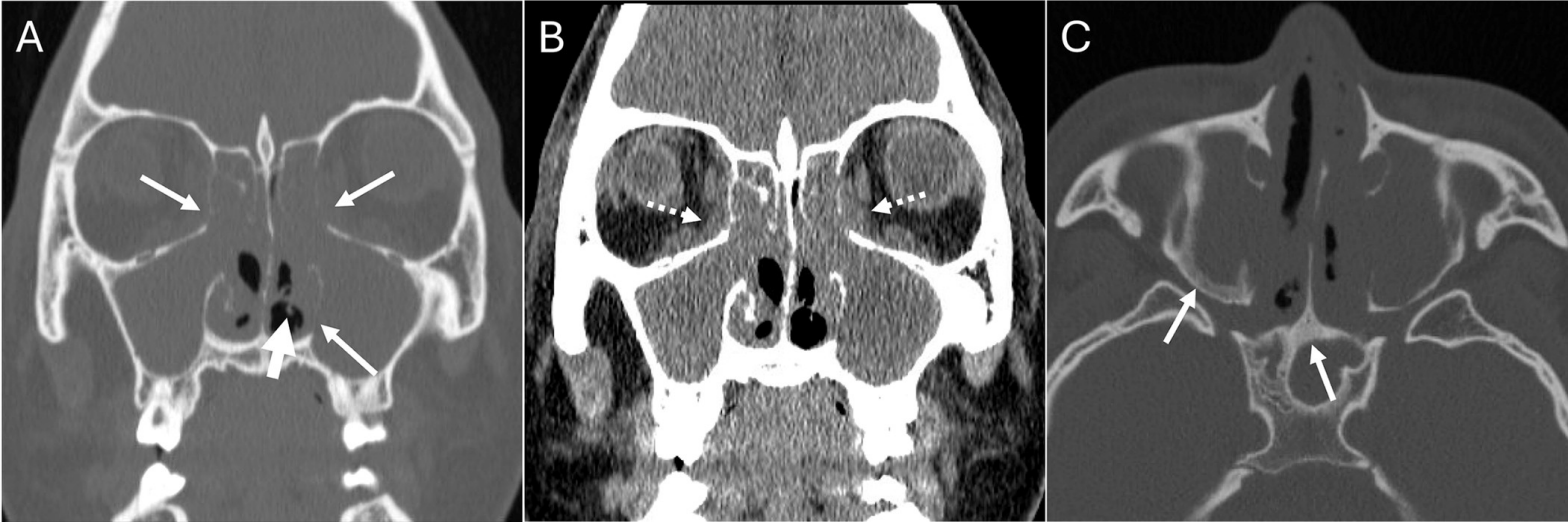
Granulomatosis with polyangiitis (GPA). Coronal bone (A) and soft tissue (B) window non-contrast CT of the sinuses in a 22 year-old male shows typical pan-sinus soft tissue opacification and nasal strands (thick arrow, A), foci of osseous erosion (thin arrows, A) and soft tissue extension into the adjacent orbits (white dashed arrows, B). Axial bone window (c) shows sclerosis of sinus walls (arrow) typical of chronic inflammatory disease and particularly GPA. Both radiologists agreed on the diagnosis of inflammation and GPA, consistent with the clinical diagnosis.
